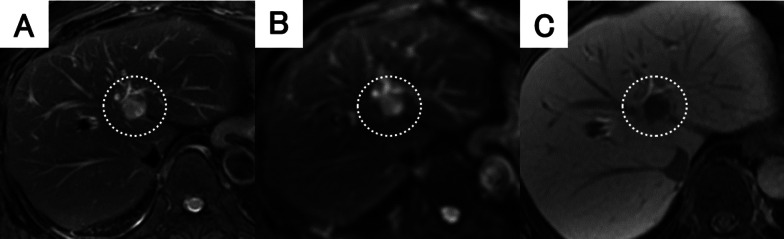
Findings of ethoxybenzyl magnetic resonance imaging (MRI). A The tumor had high signal intensity upon T2-weighted imaging. B Diffusion-weighted imaging showed high signal intensity of the tumor. C The tumor showed high intensity during the early phase and loss of primovist® uptake during the hepatocyte phase
